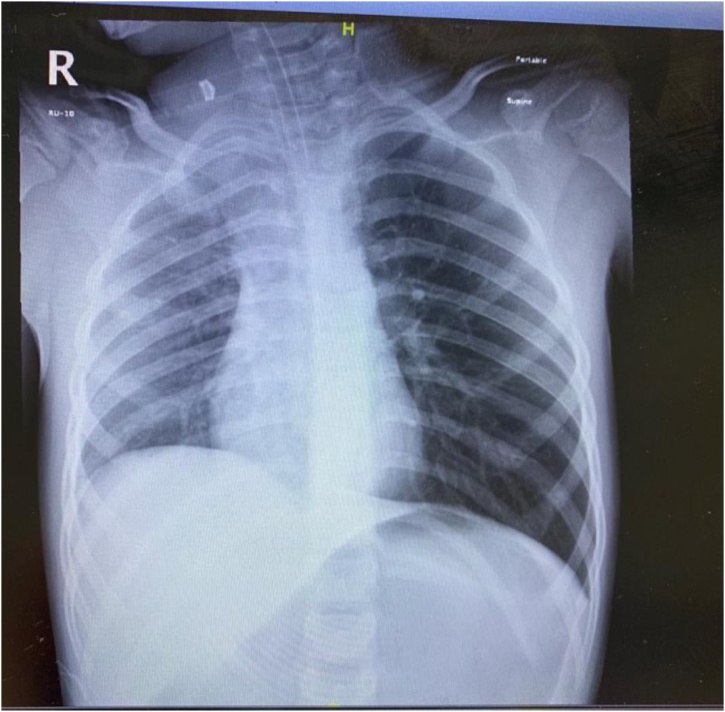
Chest x-ray after intubation showing hyperlucent left hemithorax with mediastinal shift to the contralateral side and flatting of ipsilateral hemidiaphragm.
